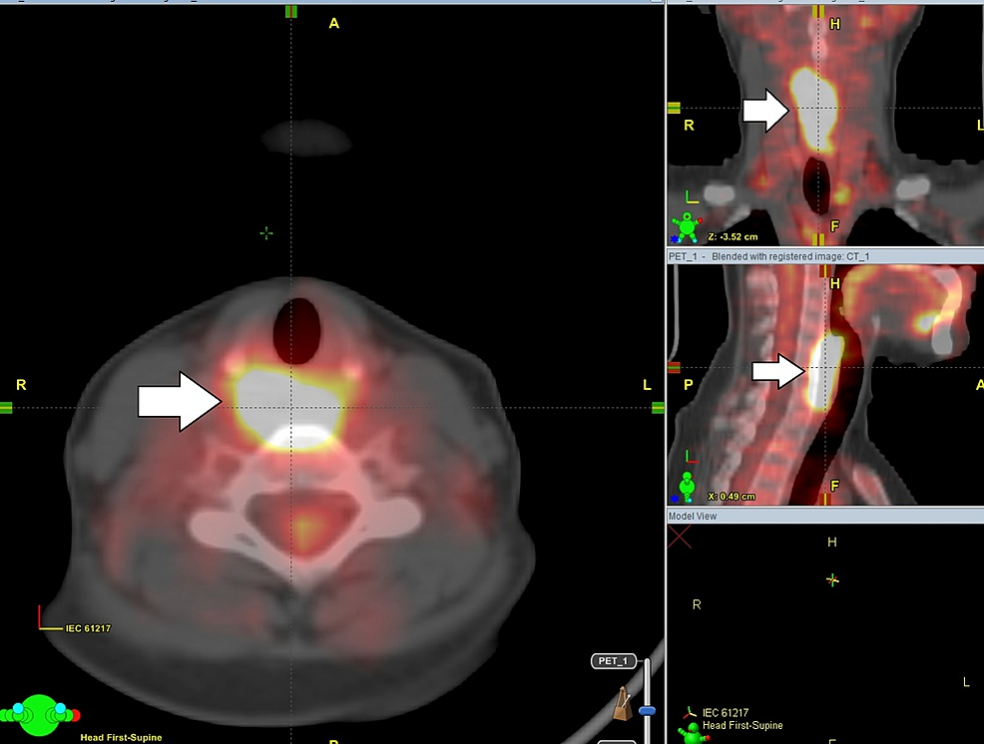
Positron emission tomography imaging The lesion indicated by the arrows is in the hypopharynx, starting from the right side of the oropharynx and extending to the proximal esophagus (SUV max: 8.5).
